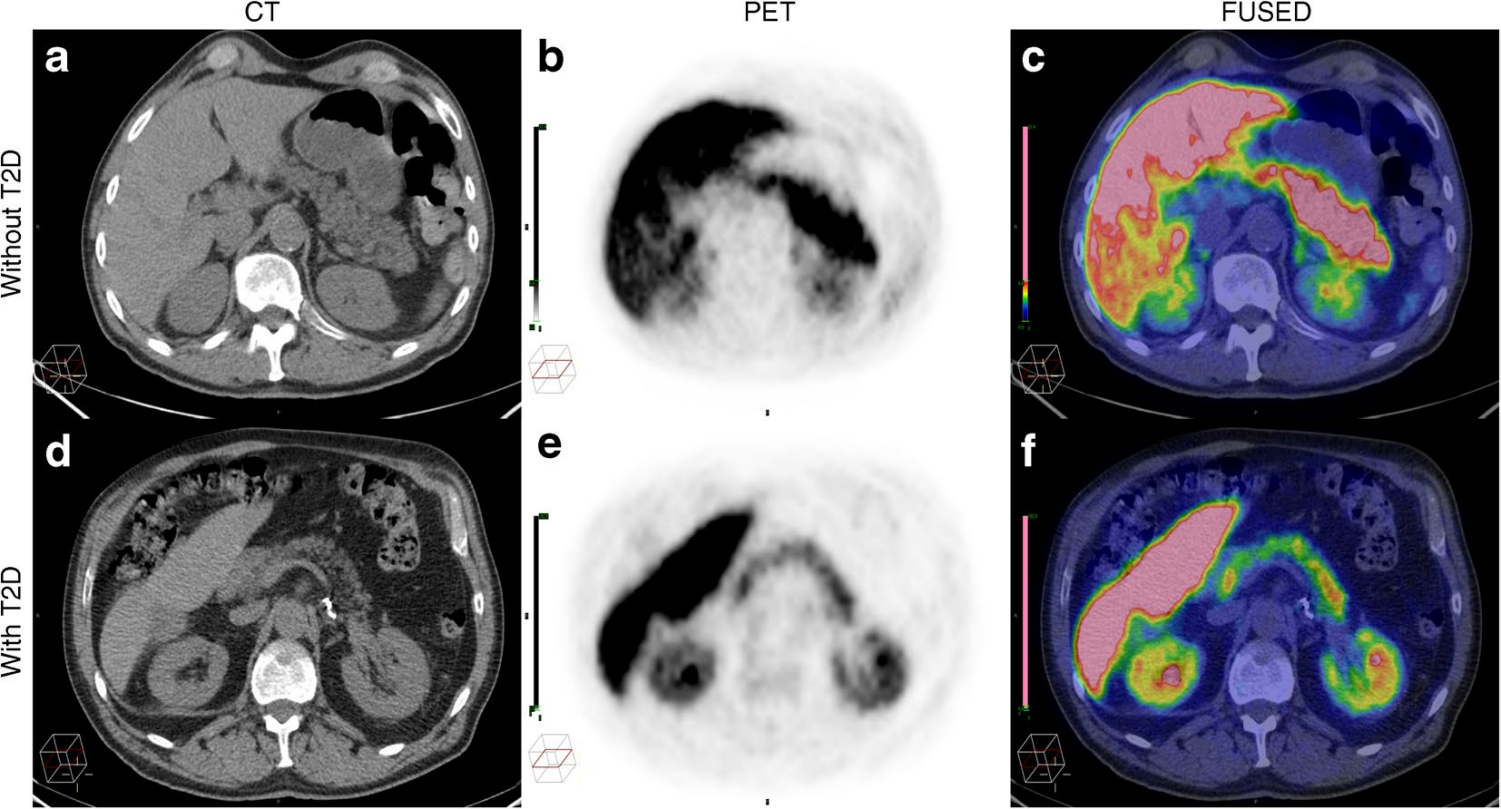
Images showing non-invasive imaging in two individuals, one without type 2 diabetes (a–c) and one with type 2 diabetes (d–f), using ^11^C-HED-PET/CT and CT. Distinct uptake of ^11^C-HED in the pancreas was seen in all examined individuals. Transverse CT (a, d), PET (b, e) and fused PET/CT (c, f) images in representative individuals. PET images are normalised to SUV = 6.6. T2D, type 2 diabetes
